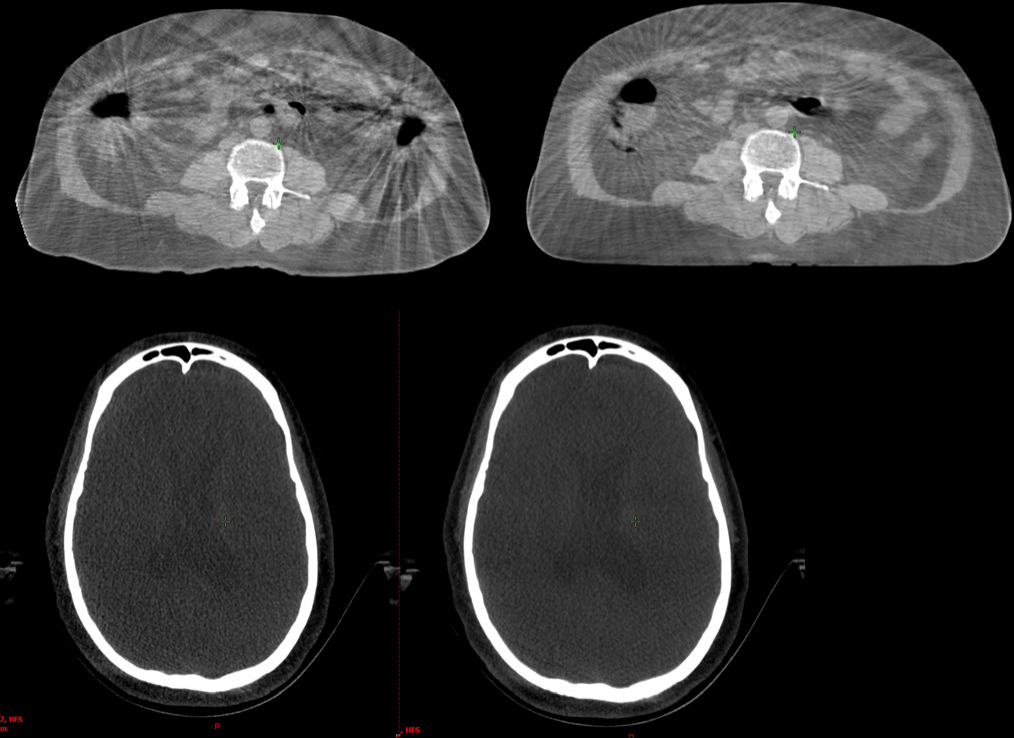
Abdomen scan taken with Varian default pelvis CBCT protocol (top left) and scan taken with Abdo/Pelvis_HD (top right). Head scan taken with Varian default Head CBCT protocol (bottom left) and scan taken with Head_SRS (bottom right). Both Abdo/Pelvis_HD and Head_SRS increased the imaging dose and improved image quality and the radiographers’ confidence to perform online image registration.
